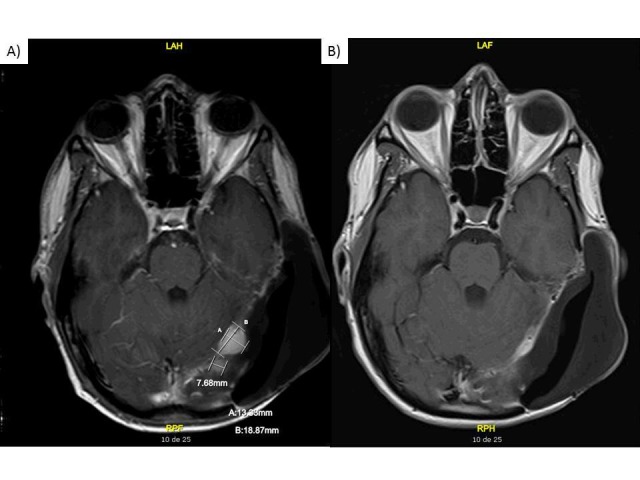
MRI images while the patient was on crizotinib Axial T1 post-contrast (gadolinium) MRI from A) initiation of crizotinib and B) seven months later.
